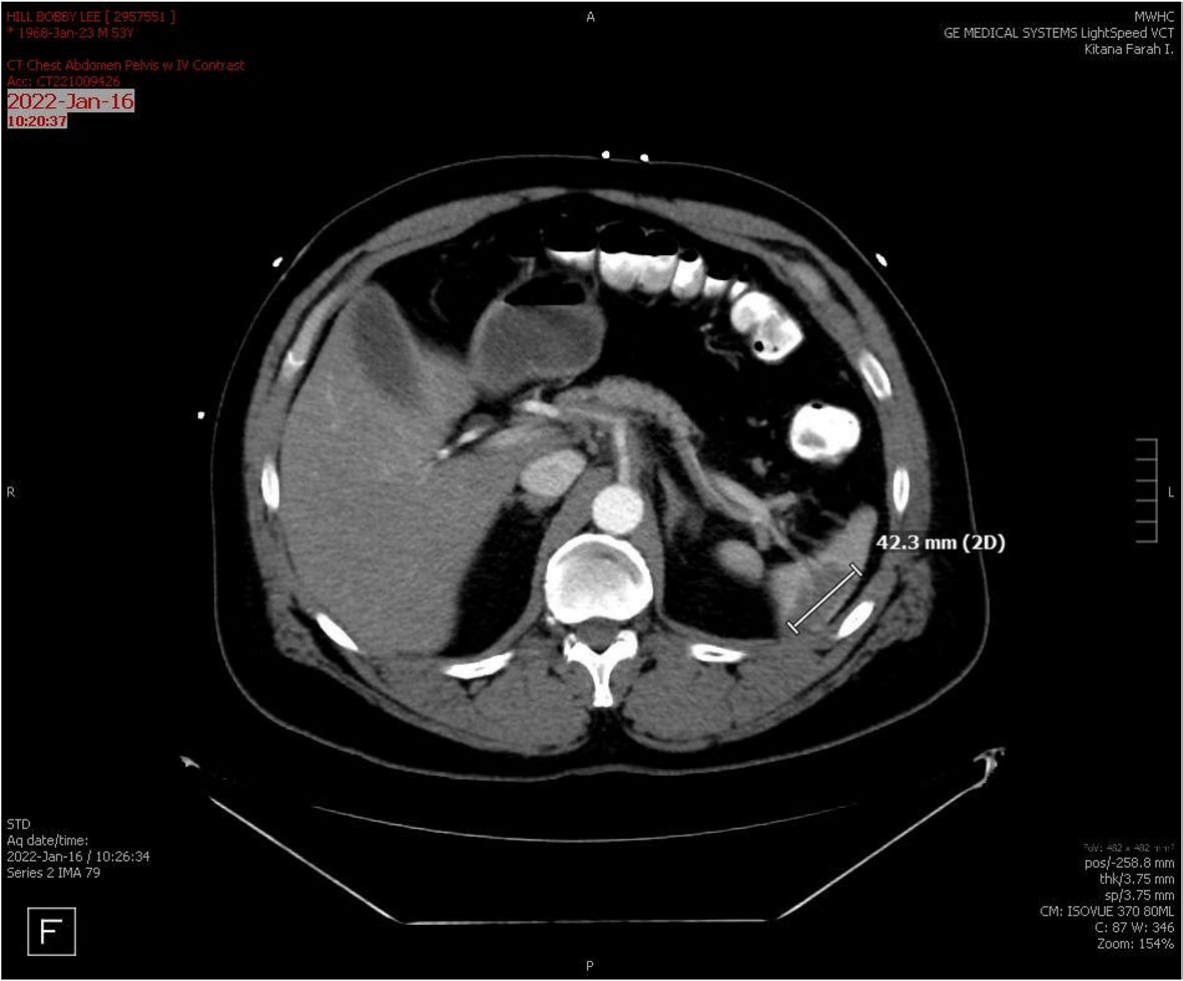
CT Abdomen – splenic infarction, hypoechoic lesion measured at 4.2 cm in length
